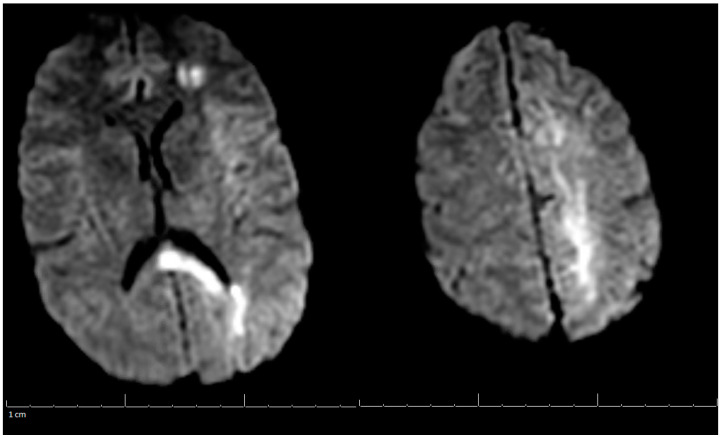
MRI of the Brain without contrast (DWI) (Magnetic Resonance Imaging (MRI) is a technique that uses magnetic fields and computer generated radio waves to create images of organs and tissues in your body. Magnetic Resonance Venography (MRV) uses magnetic resonance technology and intravenous contrast dye to visualize the veins. Diffusion-Weighted Imaging (DWI) is a form of MRI that uses the diffusion of water molecules to generate images).
